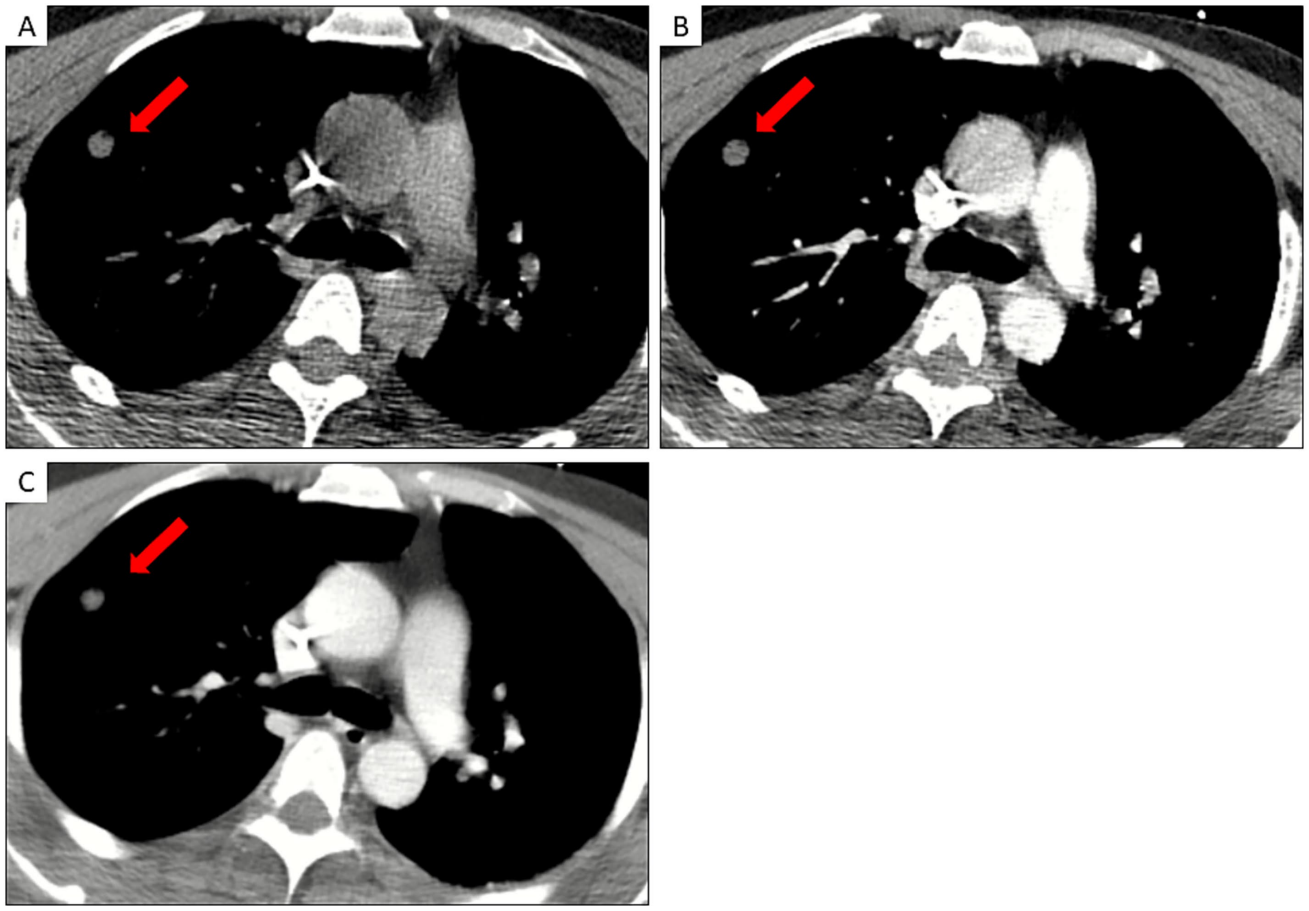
24-year-old male patient. (A) thin-layer CT mediastinal window of the anterior segmental nodular shadow in the upper lobe of the right lung; (B,C) CT contrast-enhanced arterial and delayed phases with no change in CT values of the anterior segmental nodule in the right upper lobe of the lung.
